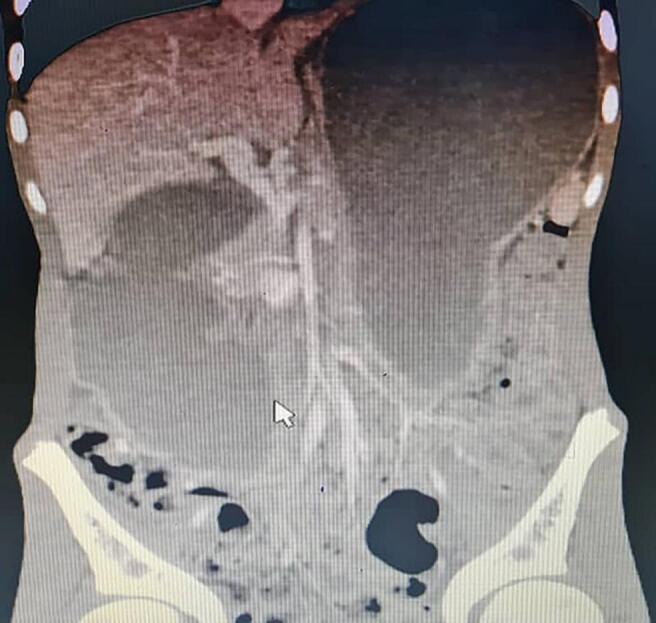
The initial CT scan shows the obstructed view.
